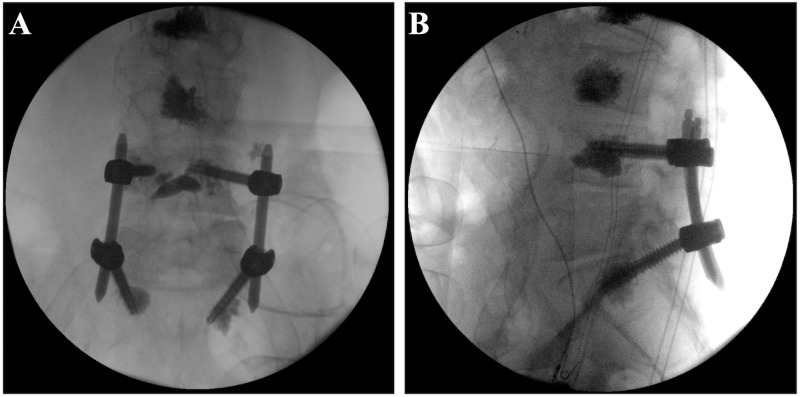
Intraoperative AP (A) and lateral (B) plain films of the lumbosacral spine, demonstrating optimally placed bilateral L4 and S1 PMMA-augmented pedicle screws.
